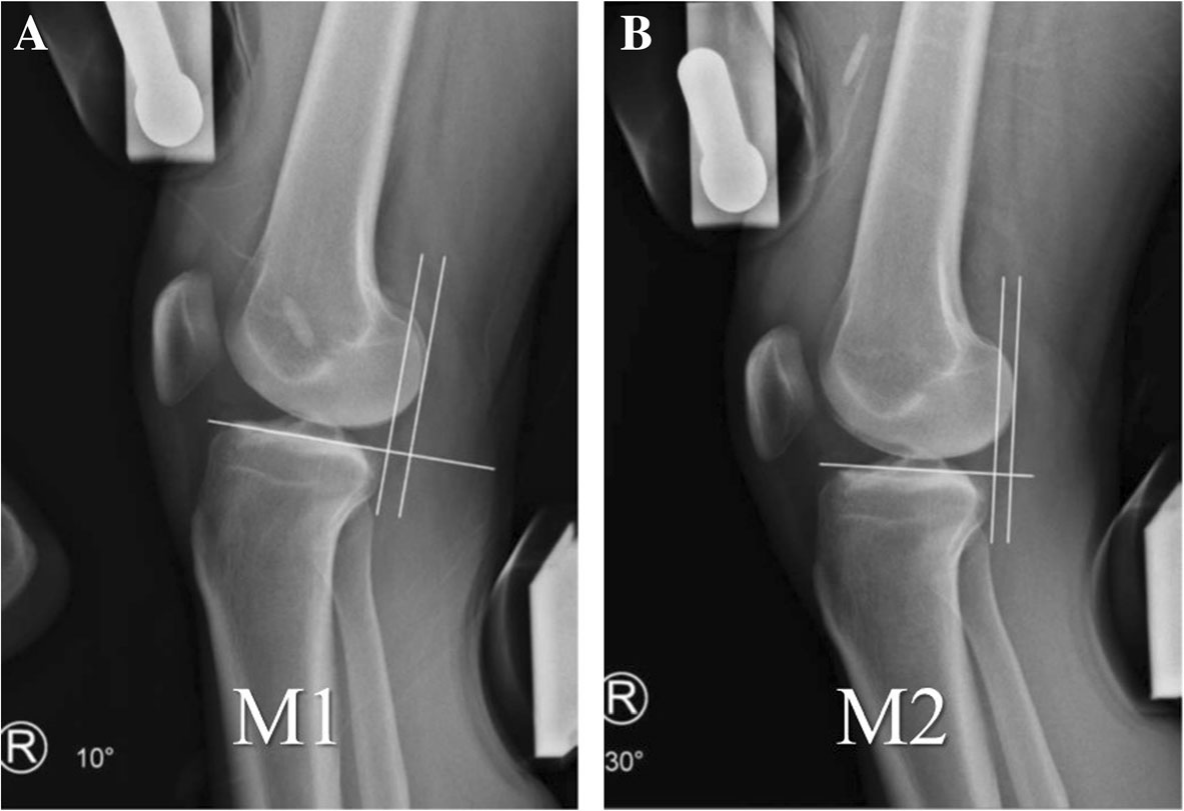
Stress radiography using Telos stress device with knee flexion 10° (a) and 30° (b). A tangent line to the posterior contour of the medial tibial condyle was drawn perpendicular to the medial tibial plateau. Distance between the line and the posterior aspect of the superimposed posterior femoral condyles was measured
